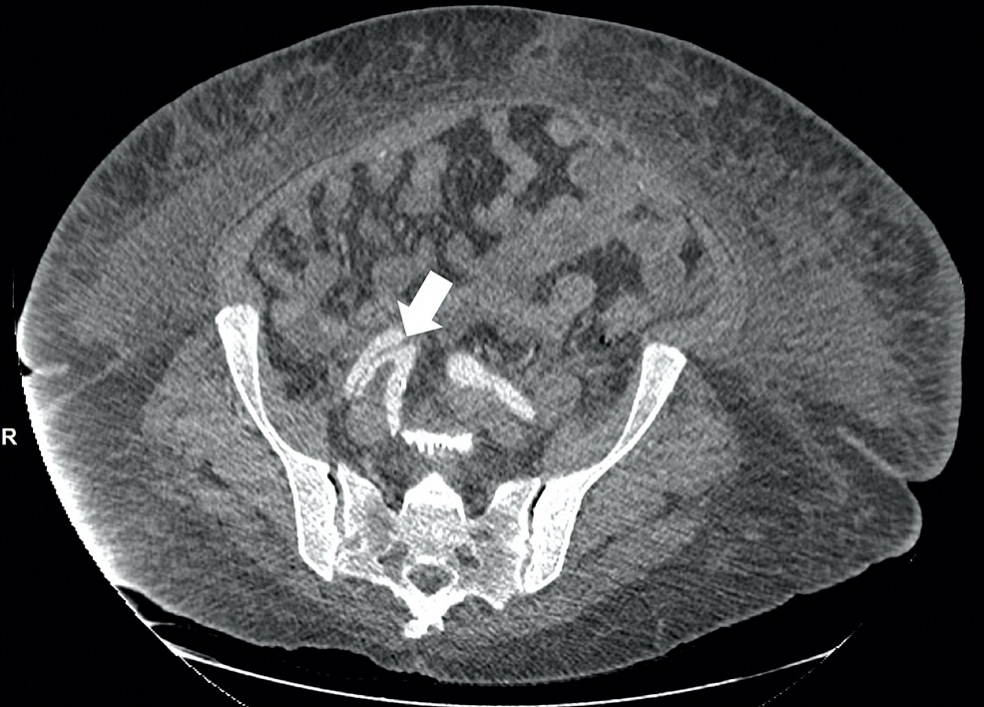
Computed Tomography Angiogram (abdomen and pelvis). The white arrow shows dissection extending into the right external iliac artery.
